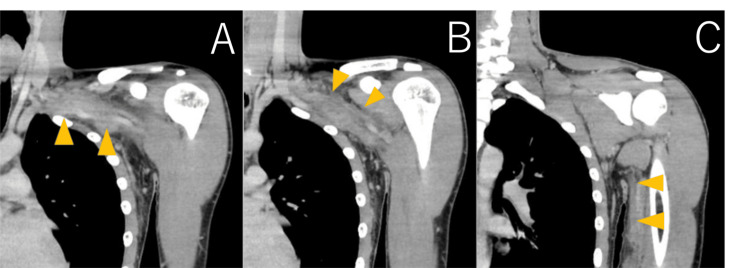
The contrast-enhanced computed tomography (CECT) images (A-C). CECT showing thrombotic occlusion of the left brachial vein extending up to the confluence with the internal jugular vein.
